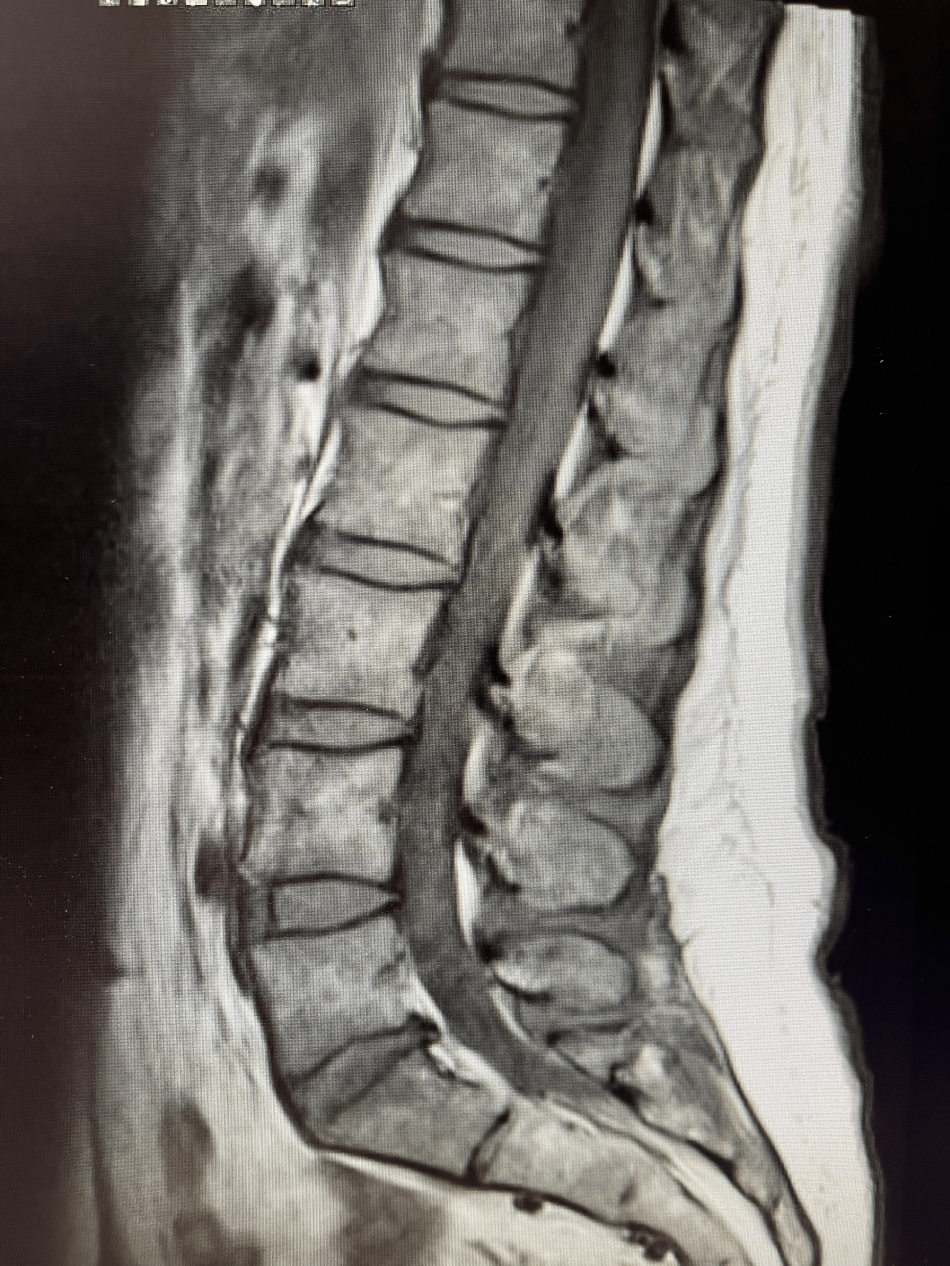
Sagittal T2-weighted MRI of the lumbar spine Sagittal T2-weighted magnetic resonance imaging (MRI) of the lumbar spine demonstrating multilevel degenerative disc and facet joint disease with mild spinal canal stenosis, without evidence of cauda equina inflammation, nerve-root enhancement, or acute demyelinating pathology.
